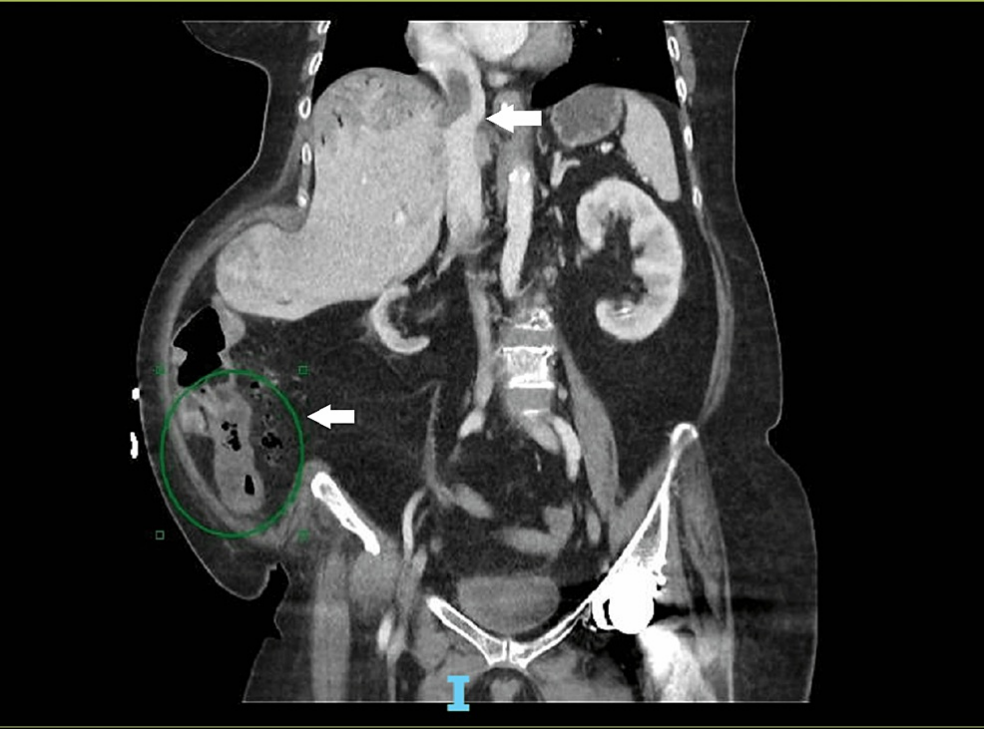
Contrast-enhanced CT (coronal view) in the portal venous phase done on the day of admission showing thrombus in the right hepatic vein extending into the inferior vena cava (IVC) and downstream terminal ileum is collapsed and associated mural oedema.
